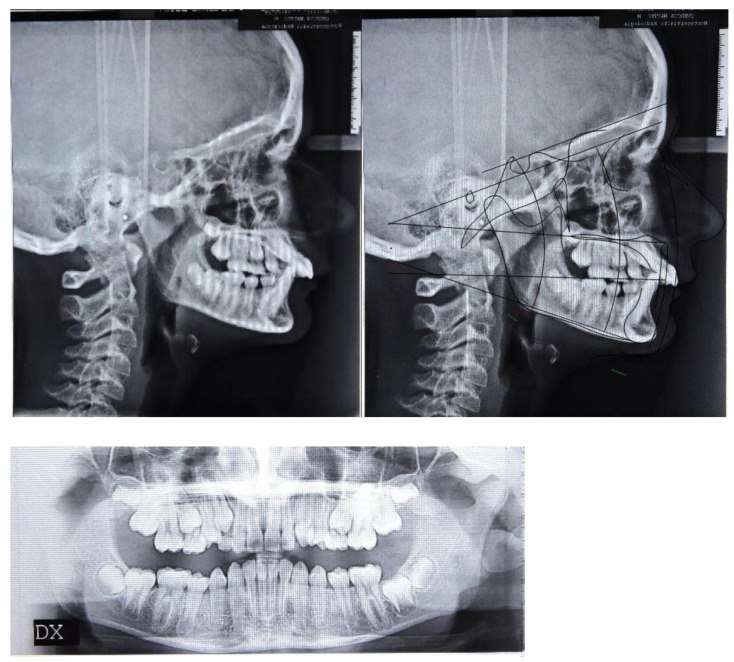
Pre-treatment intraoral, extraoral photographs and radiographic examination.
